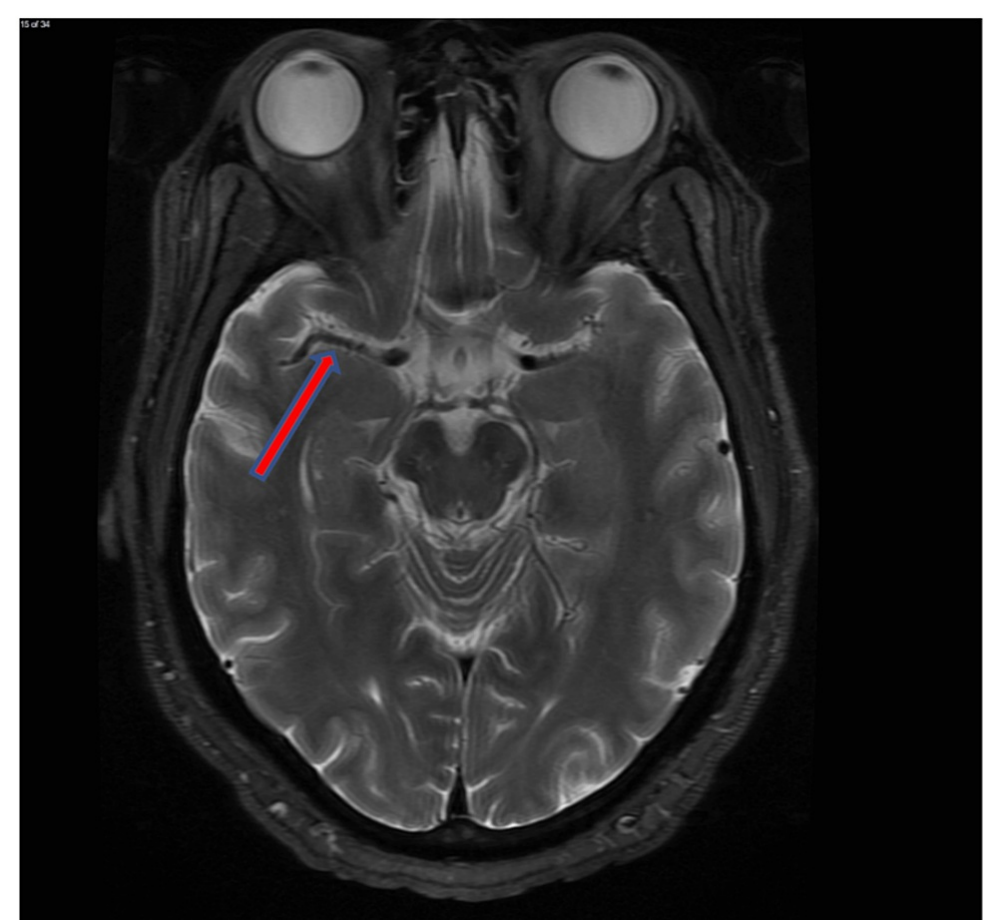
T2 weighted MRI from 2 years earlier shows patent right middle cerebral artery (red arrow).
